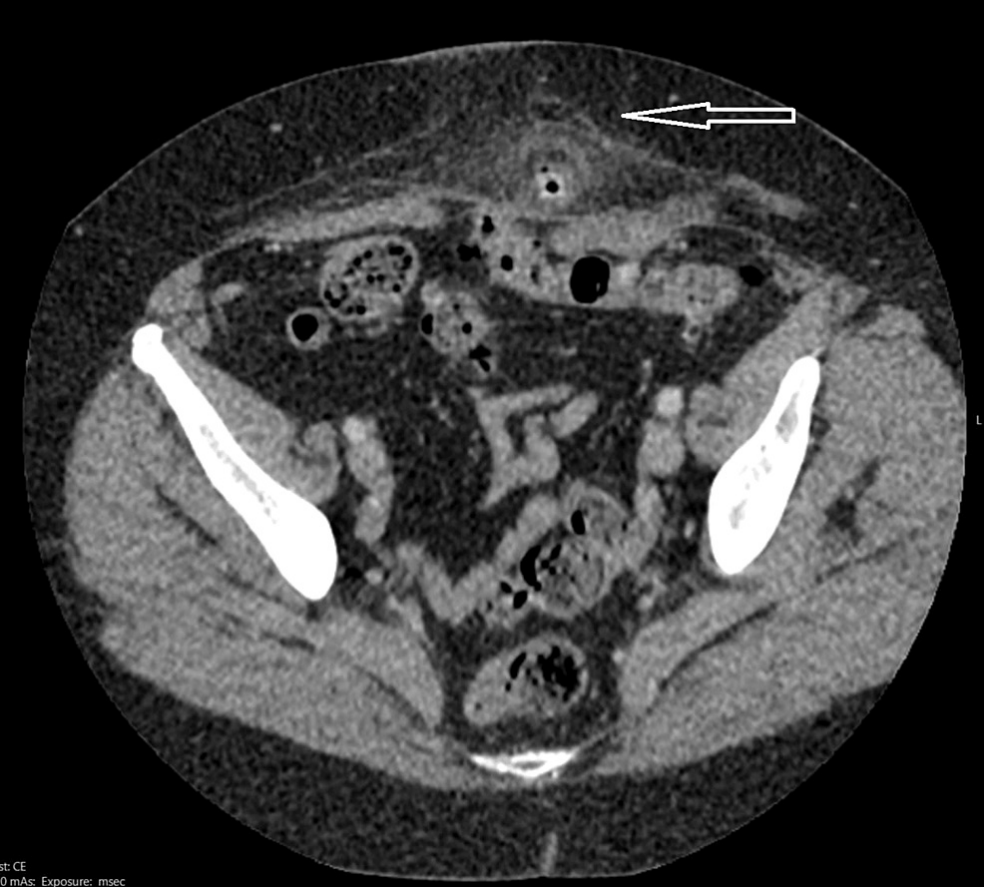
Axial view CT scan of the abdomen showing ventral hernia sac containing sigmoid diverticulitis.
